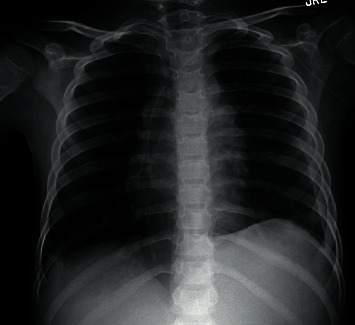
Chest radiograph before discharge demonstrating resolved pulmonary edema.
